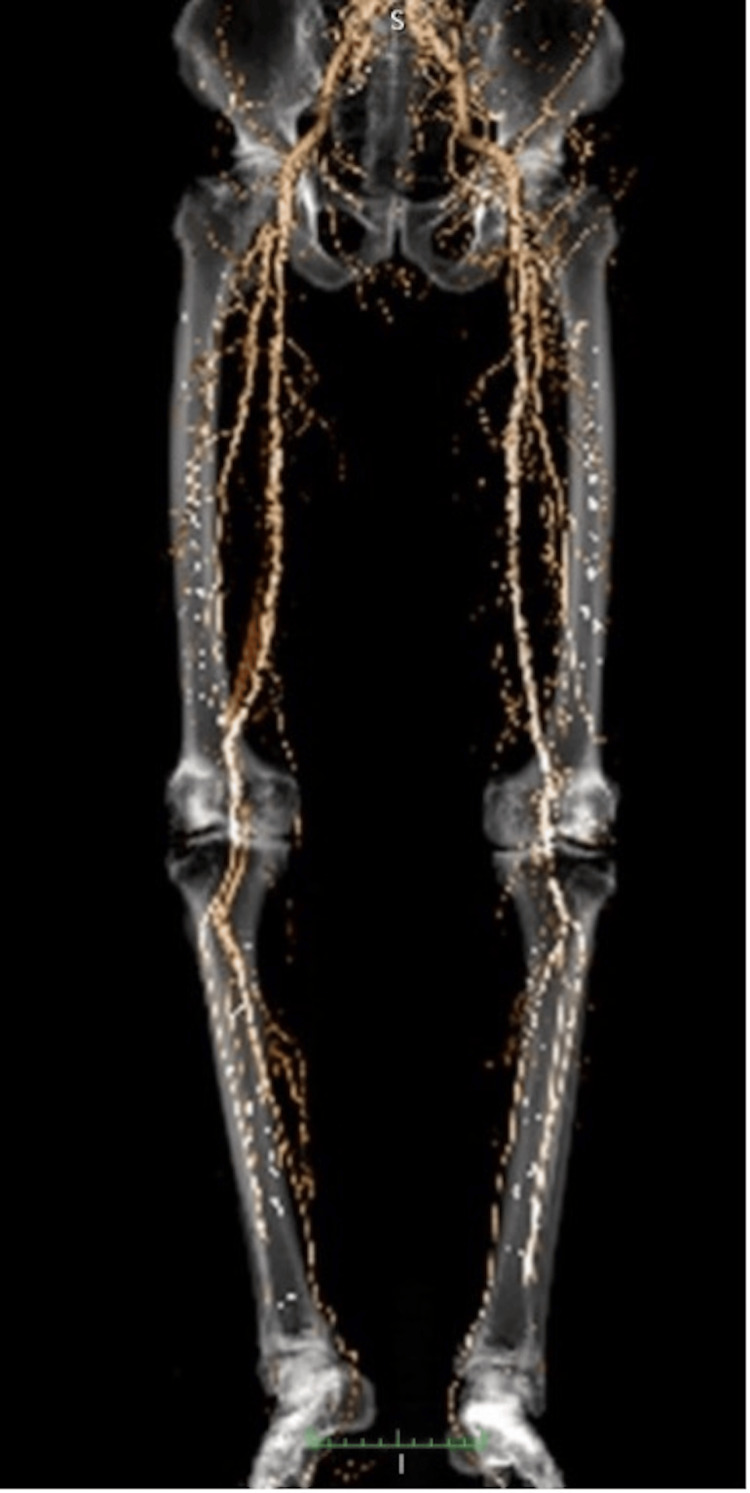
Preoperative computed tomography angiogram revealed severe calcification and occlusion from the common femoral artery to the femorotibial popliteal artery.
